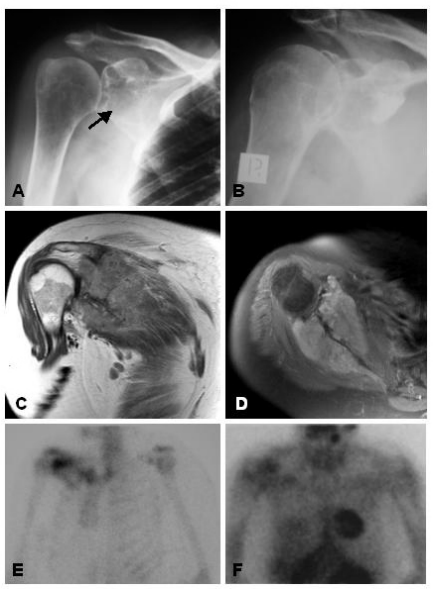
A Plain radiograph of the right shoulder, showing an irregular, mixed lytic and sclerotic lesion in the glenoid (arrow), that was not appreciated by the reporting radiologist. B Arthrogram performed prior to hydrodilatation. C Coronal TSE post-contrast MR image, showing a diffusely enhansive scapular lesion extending into the inferior aspect of the gleno-humeral joint. D Axial TSE post-contrast MR image showing diffuse enhancement of the tumour extending on either side of the scapular blade with bony destruction. E Bone scan showing increased uptake in the area of the lesion on the delayed static image. F Thallium functional scanning showing retained thallium activity in the glenoid region at 4 hrs. Subsequent biopsy was consistent with Ewing's sarcoma.
